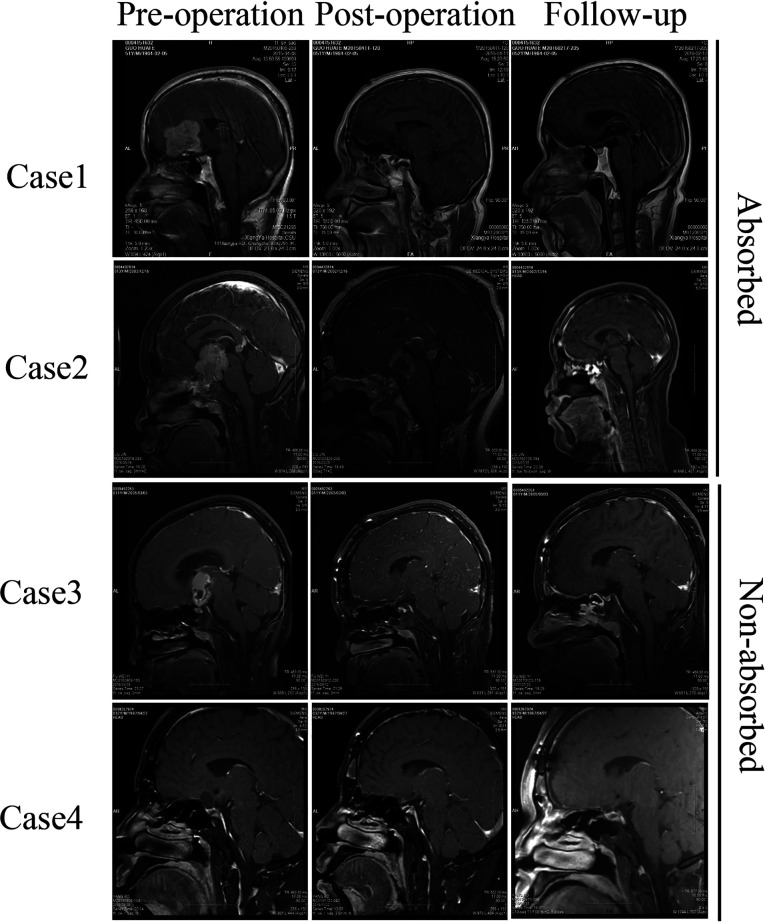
Pre/post- operative and follow-up MRI study. The pre-operative MRI showed the lesion at the anterior skull base and the frontal sinus. The post-operative MRI showed the open frontal sinus filled with gelfoam. The follow-up MRI showed that the gelfoam was absorbed or not absorbed during follow-up.
